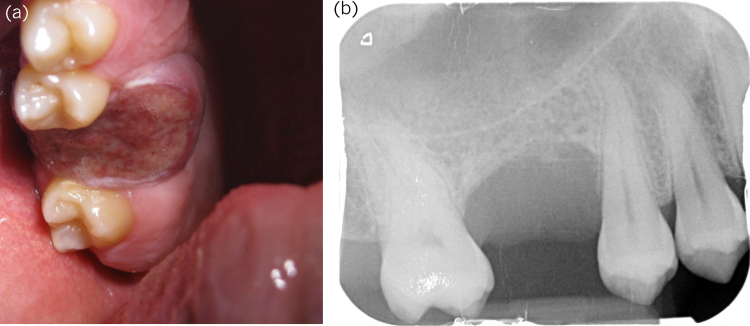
Peripheral giant cell granuloma: (a) manifesting as ulceration in the extraction socket of the upper right six; (b) periapical showing intact floor of sinus and absence of roots in area imaged.
